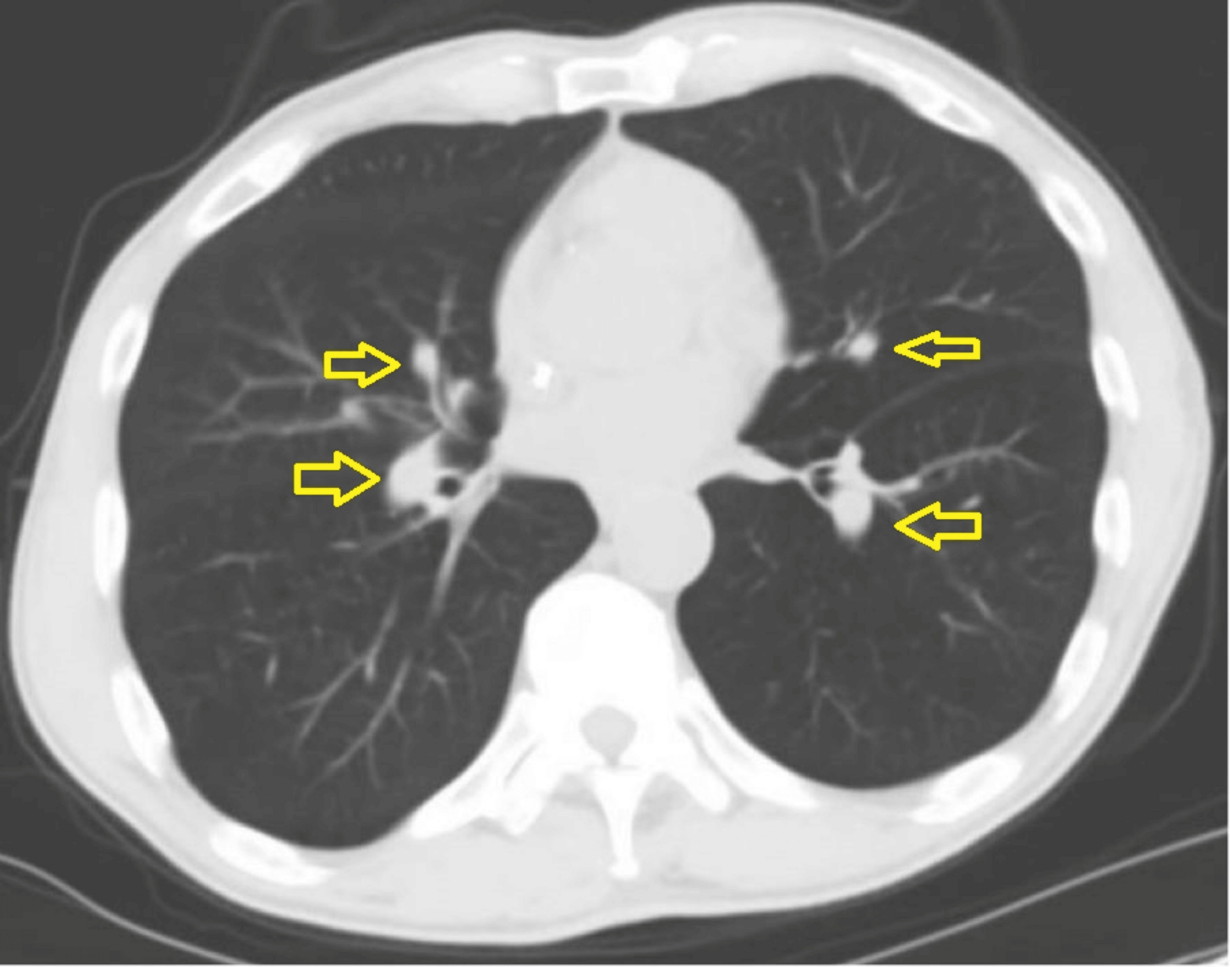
Computed tomography of the chest Arrows indicate the nodules.
